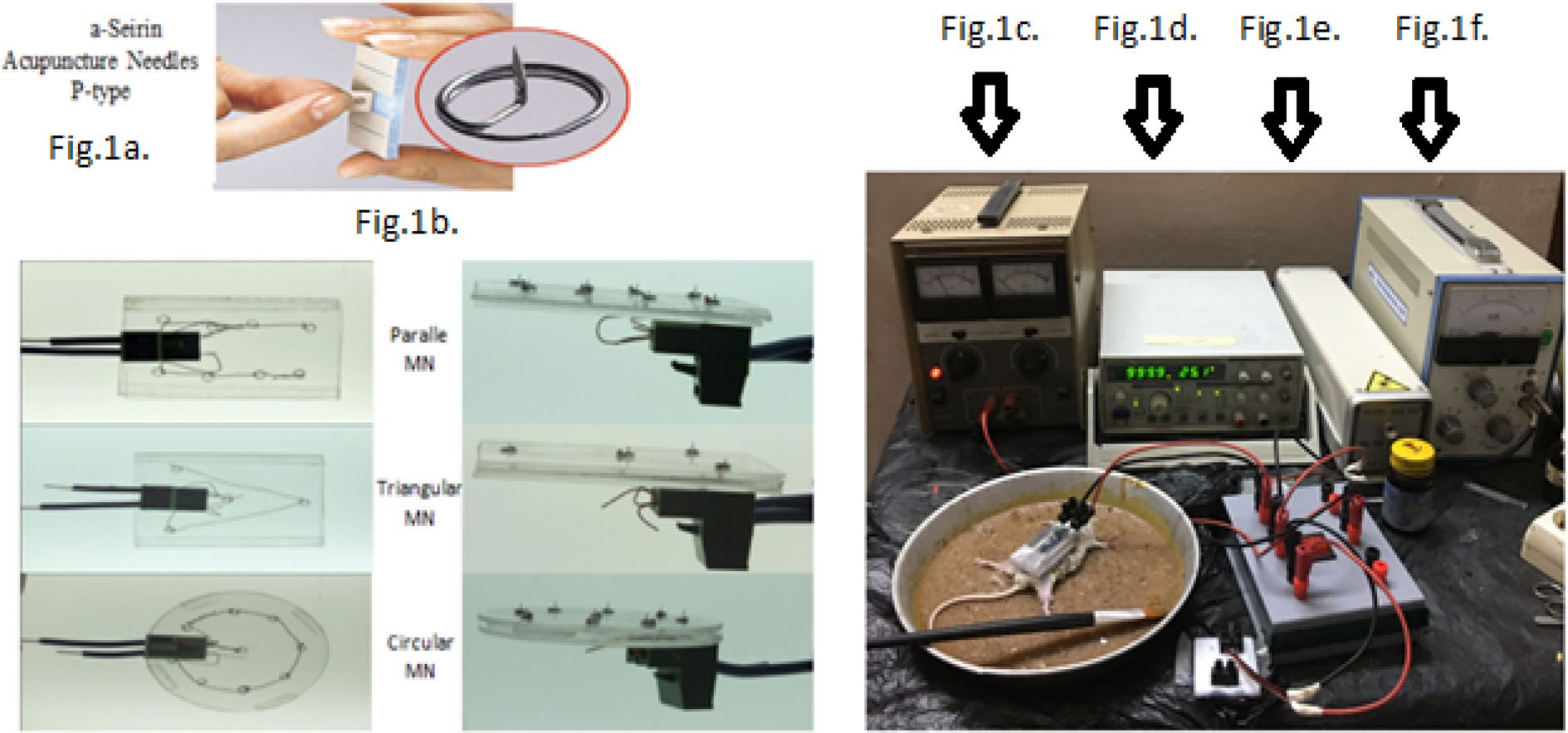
Microneedle, electroporation, iontophoresis assisted transdermal delivery of *G. macrophyllus* [MN@IP@EP@TDD *G. macrophyllus*] (a,b). (Serine type- parallel, circular and triangular) Microneedle [MN], (c). Electroporation [EP], (d). Iontophoresis [IP], (e). Laser [INRL], (f). Ultrasound [US].
